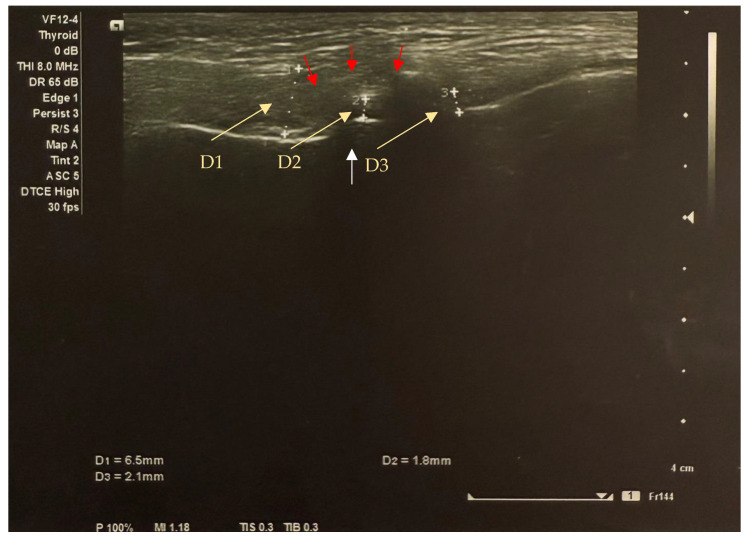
Transverse image of the left TMJ ultrasound in the closed mouth position shows anterior disc displacement (yellow arrows indicate the distance between disc thickness markers): anterior section (D1) = 6.5 mm; middle section (D2) = 1.8 mm; posterior section (D3) = 2.1mm. The white arrow shows the condyle (the condyle surface is hyperechogenic (high reflection of sound waves) and appears white on ultrasound images); the red arrows show the sutural capsule (the connective tissues represented by the joint capsule are isoechogenic (intermediate reflection of sound waves) and appear inhomogeneously grey on ultrasound images).
